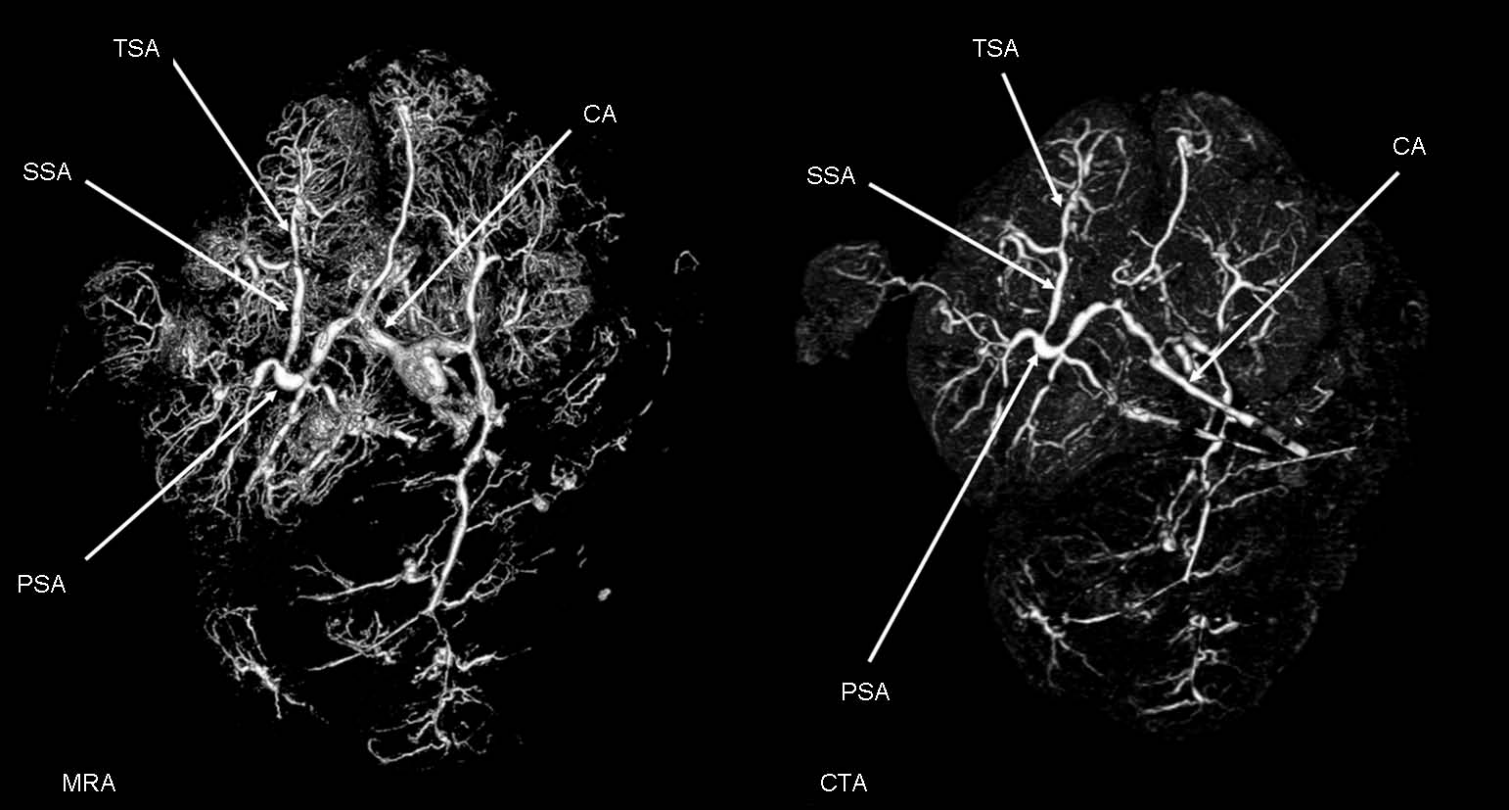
Left: MRA of human placenta; 3D reconstruction made using Osirix software viewed from the foetal side of the placenta. Key: CA, chorionic artery; PSA, primary stem artery; SSA, secondary stem artery; TSA, tertiary stem artery. Right: CTA of human placenta; 3D reconstruction made using Osirix software viewed from the foetal side of the placenta. Key: CA, chorionic artery; PSA, primary stem artery; SSA, secondary stem artery; TSA, tertiary stem artery.
